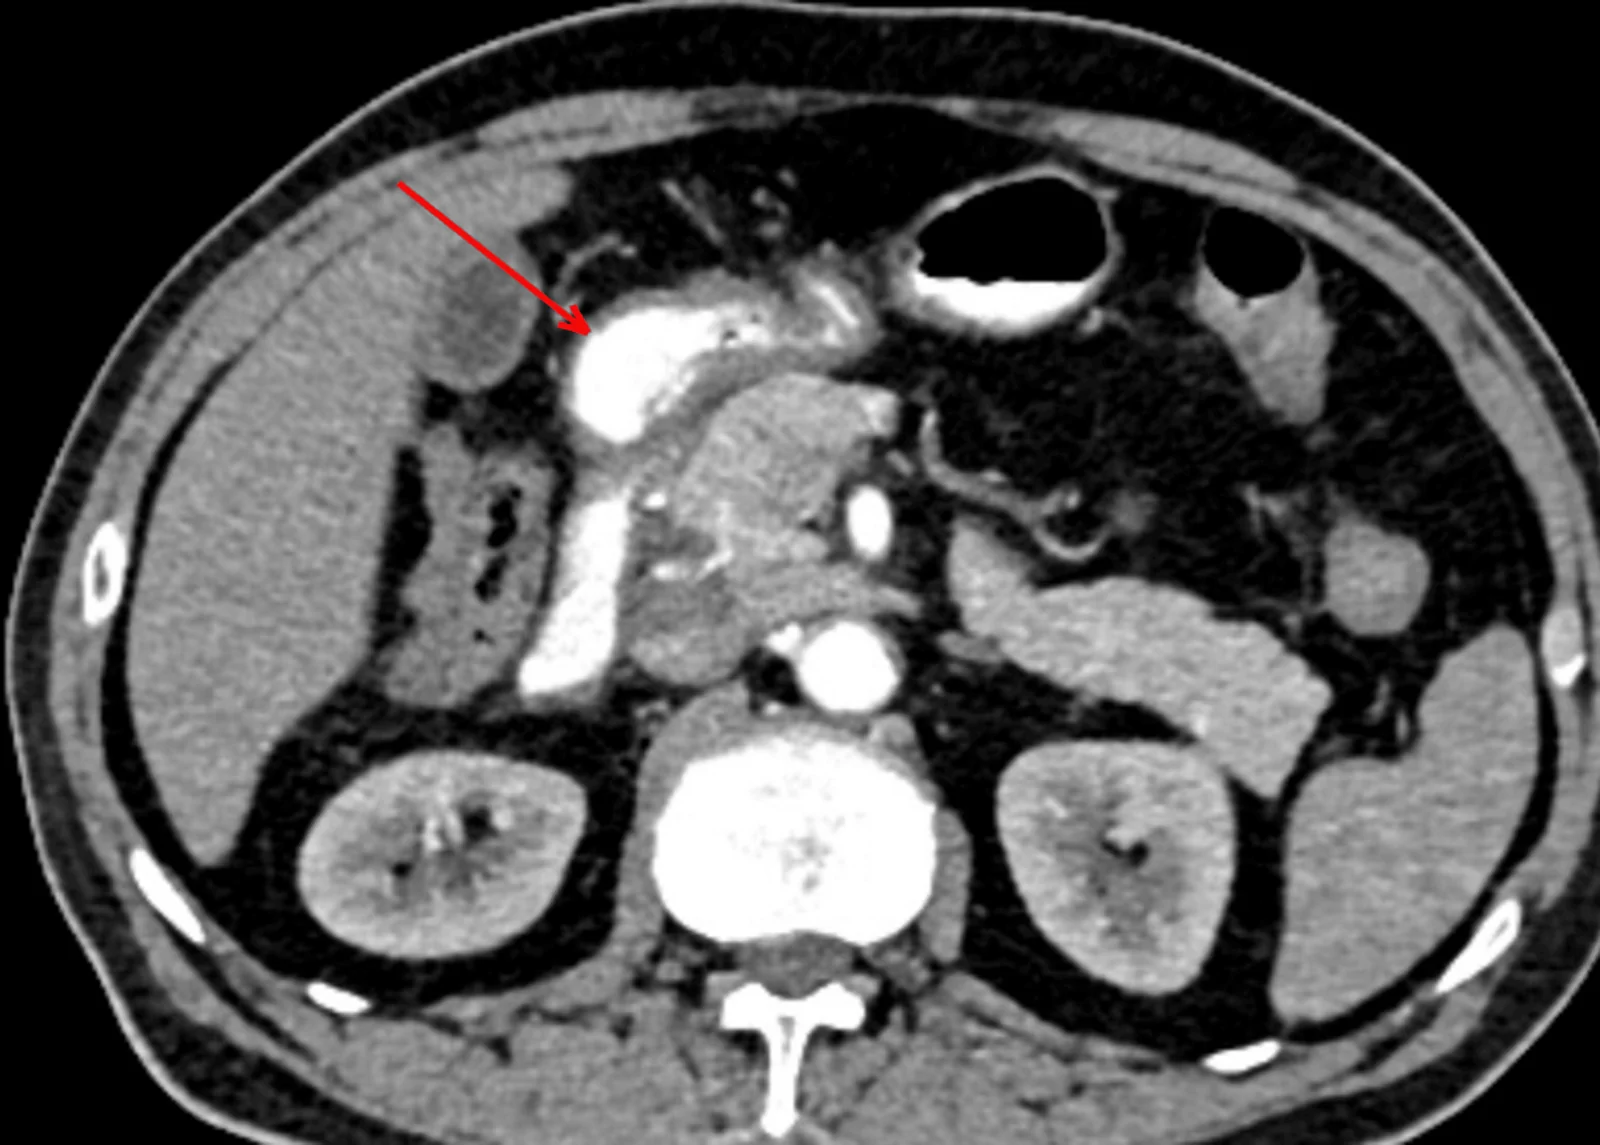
Arterial-phase contrast-enhanced abdominal CT demonstrating a bulky pancreatic head and uncinate process (arrow) without a discrete mass lesion
